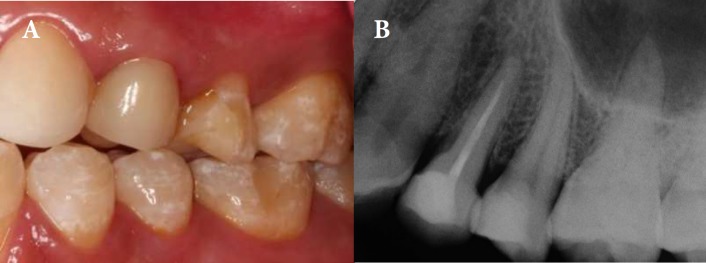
A*) Buccal view of tooth 24; *B*) Periapical radiograph one year after treatment completion
